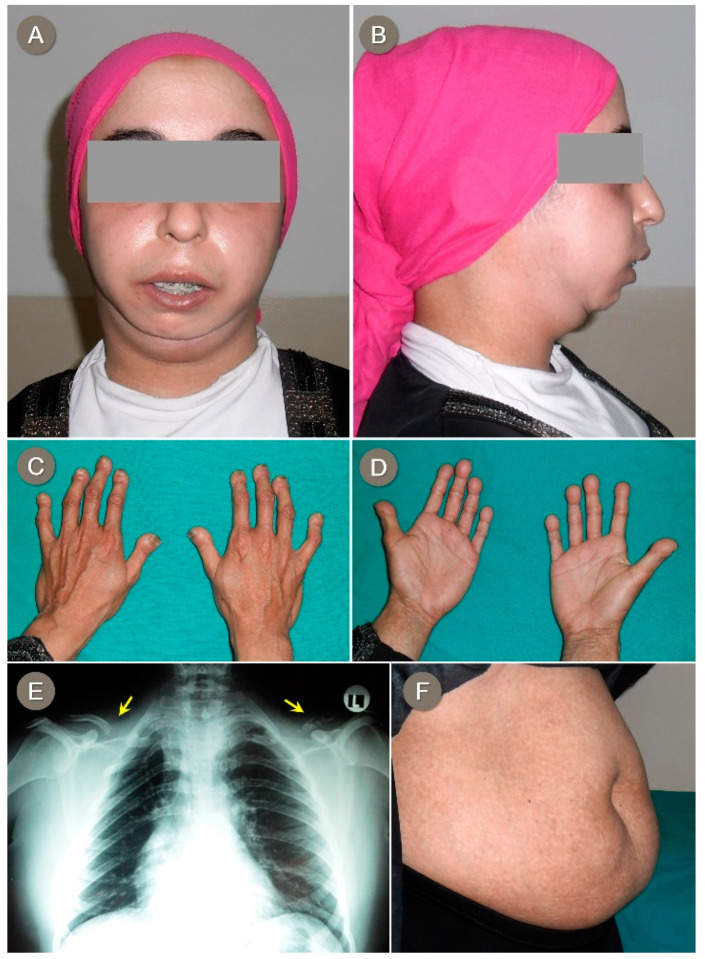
Proband 1 shows an oval-shaped, bird-like face; wide prominent eyes; full cheeks; submental obesity; arched, heavy eyebrows; a narrow, prominent nasal bridge and shallow philtrum (A); a beaked nose and severe mandibular hypoplasia (B); bulbous fingertips and nail dystrophy (C,D); marked bilateral clavicular hypoplasia evident on chest X-ray (E); and marked abdominal obesity and reticular/mottled hyperpigmentation (F).
